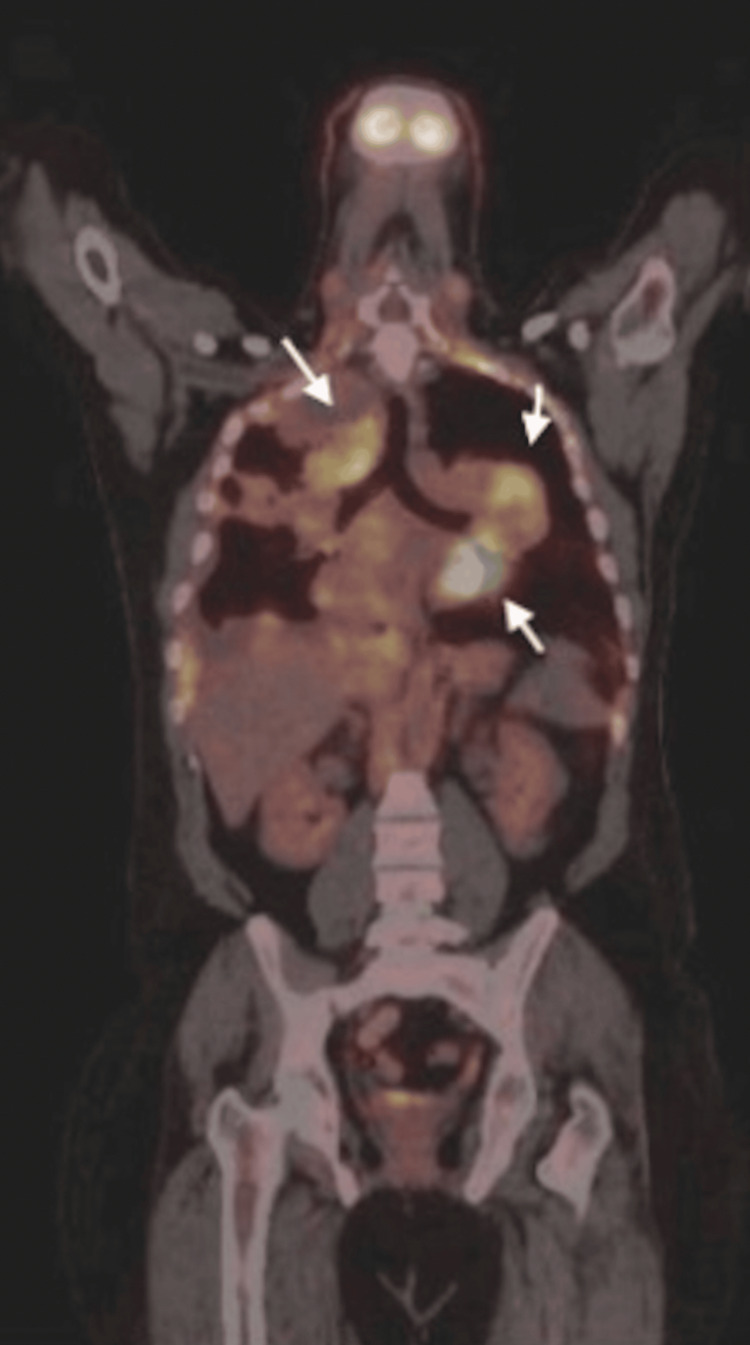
Coronal PET CT study showed multifocal FDG-18 vivid uptake in the pleural-based pulmonary soft tissue masses Positron emission tomography-computed tomography (PET CT); Fludeoxyglucose-18 (FDG-18)
